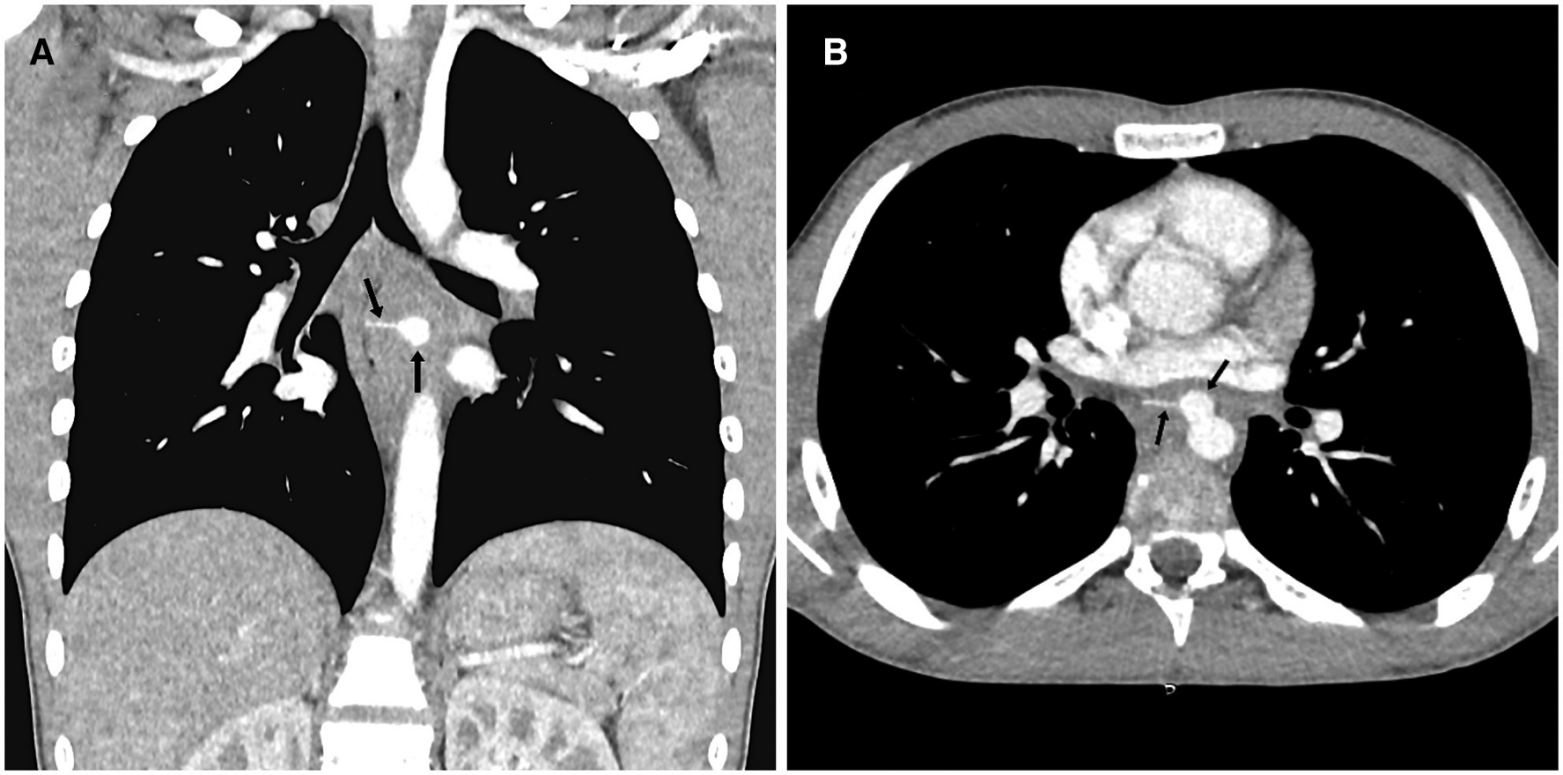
Computed tomography image with contrast.
